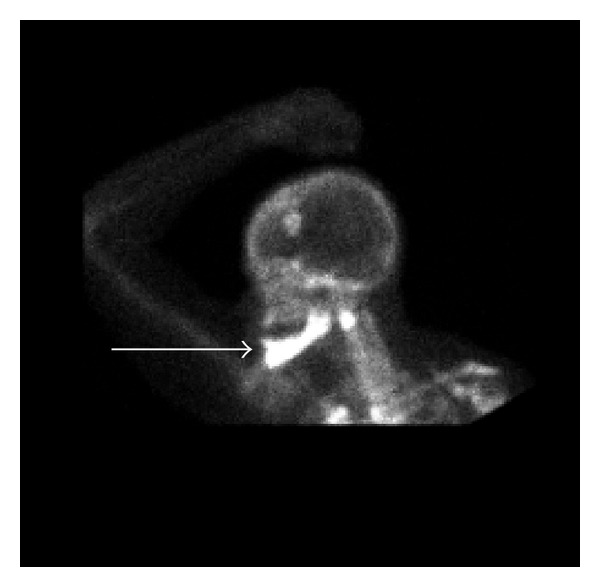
Repeat Technetium 99m bone scintigraphy. Progression of diffuse intense bony radioactivity involving most of the mandible, most likely related to diphosphonate induced mandibular osteonecrosis.
